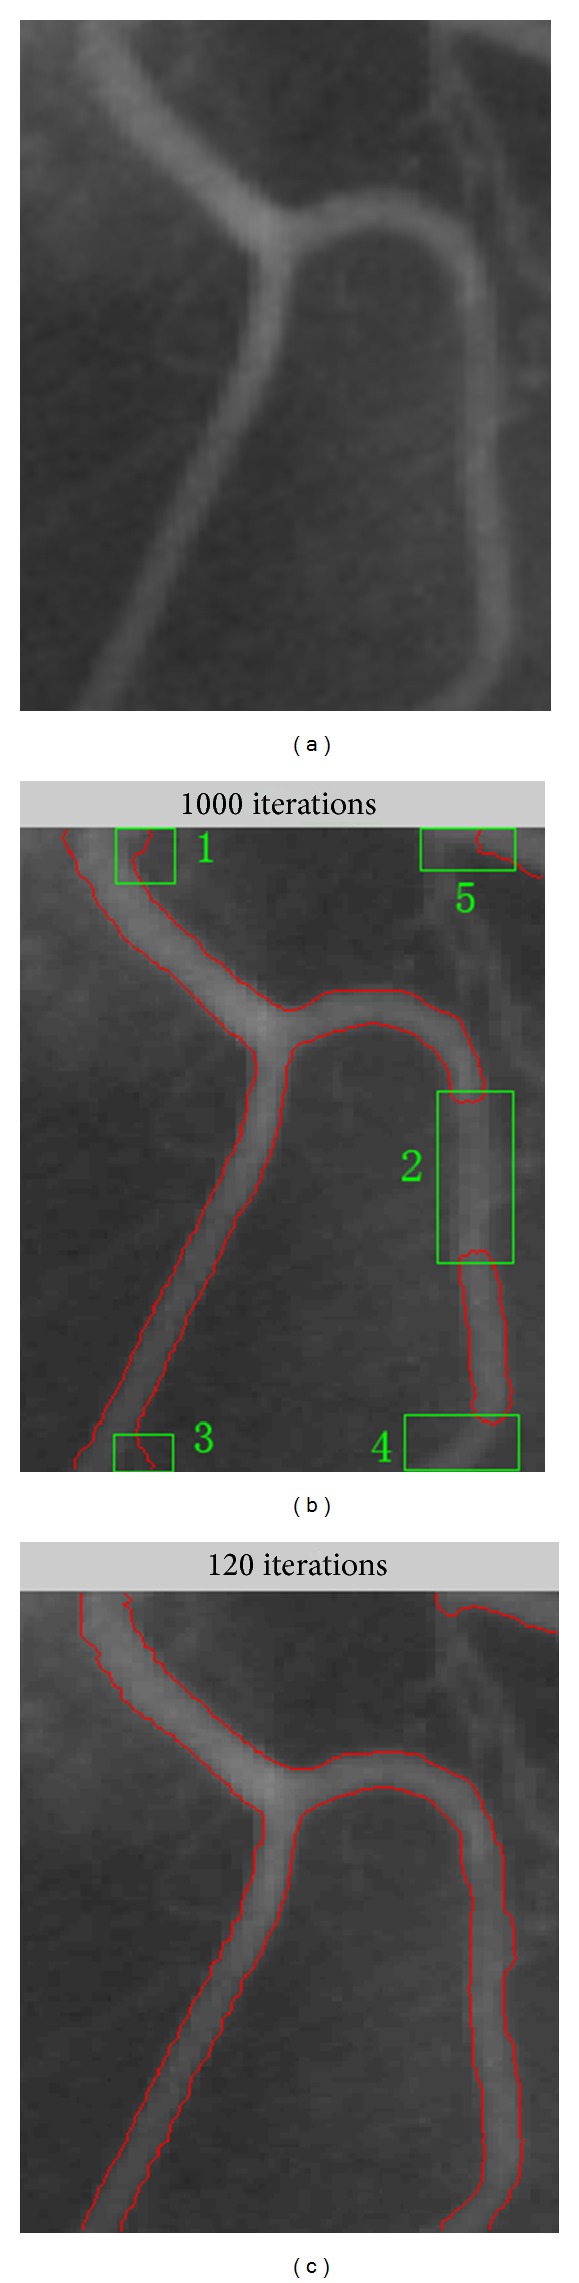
The vessel segmentation of an X-ray image with the two methods. (a) The original image. (b) The result of the ADPLS method. (c) The result of the LBF method.
